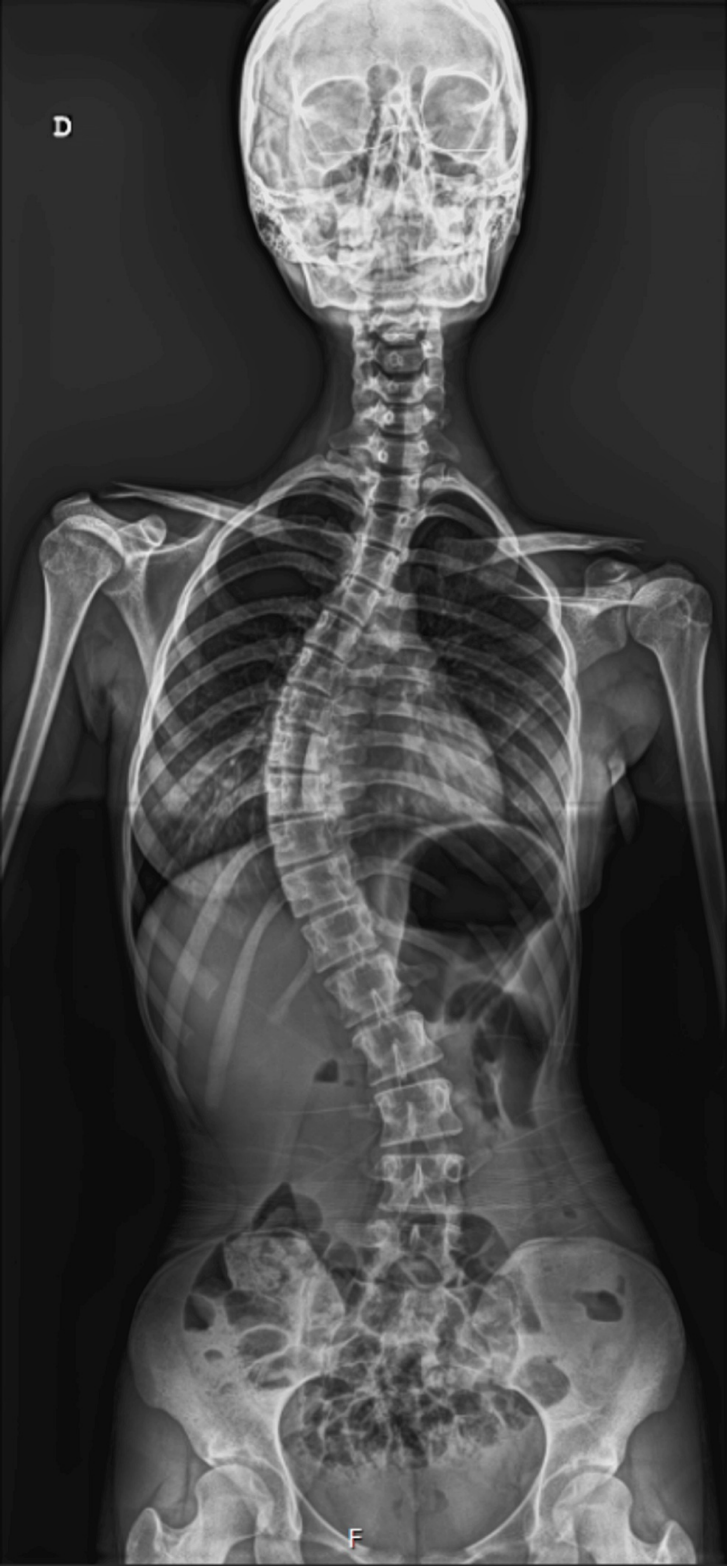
The patient's preoperative X-ray
